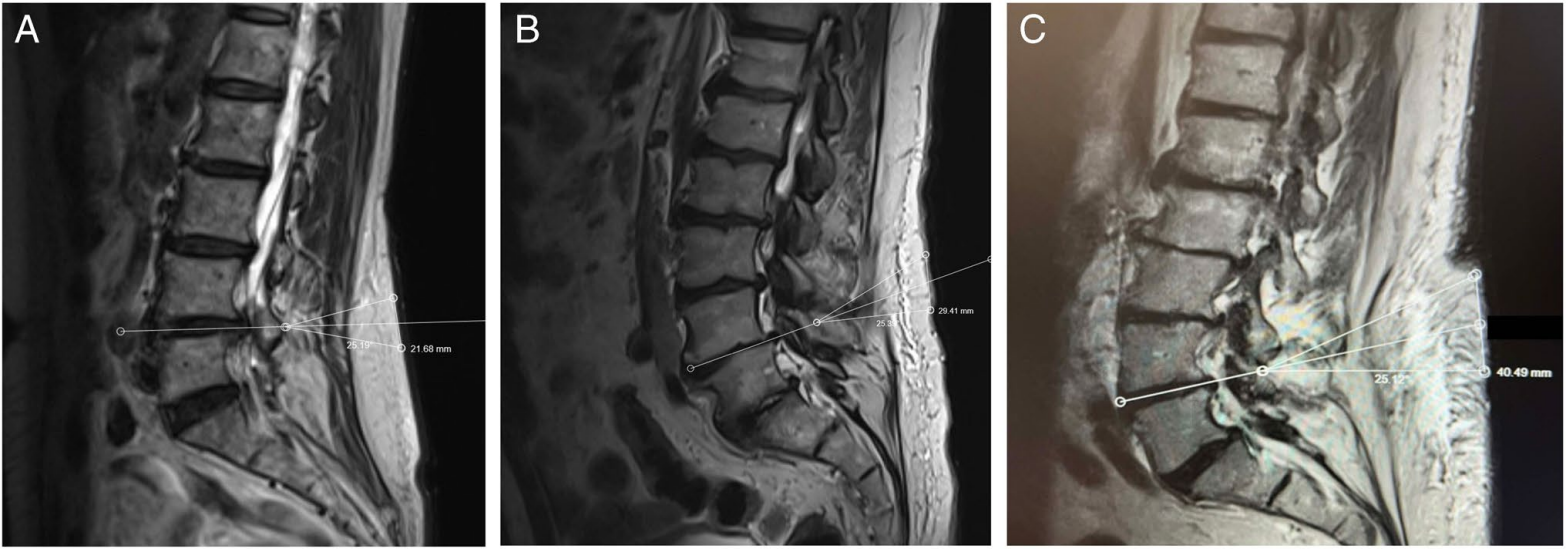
Method depicted in preoperative planning based on the T2-weighted sagittal image showing the target level and lamina of interest. The disc space line is first drawn and the 2 lines above and below the disc space line are drawn from the skin level to the target lamina that are equidistant to the disc space line. The lines converge to the target lamina at an angle of approximately 25 degrees. The distance between the 2 lines is then measured to determine the distances between the portals. Portals are typically 2 cm apart for patients with BMI of 20–25 (A), 3 cm apart for BMI 25–30 (B), > 4 cm apart for BMI > 30 (C)
